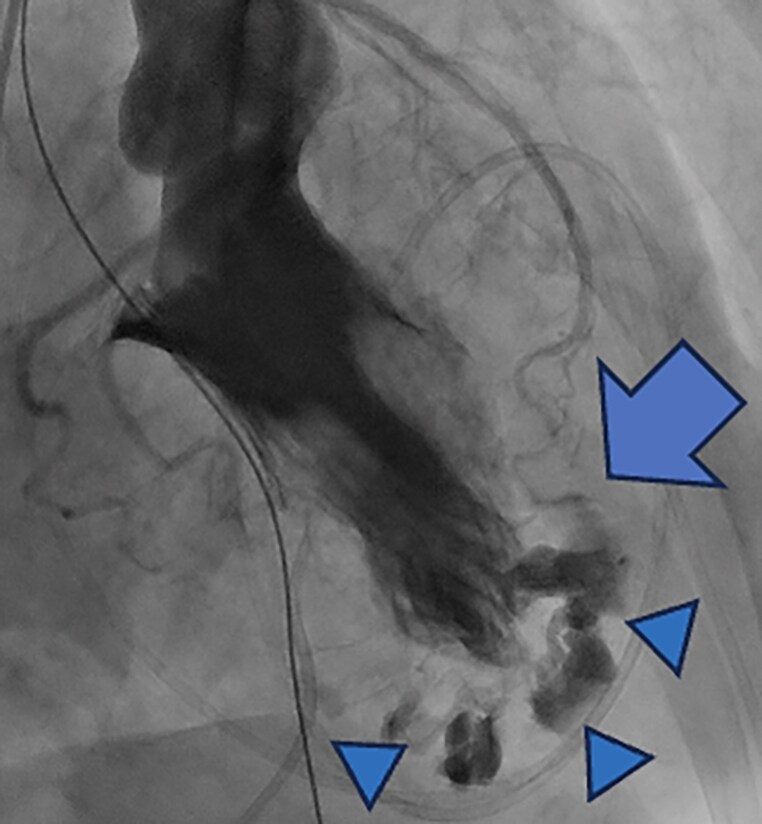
Video 2: left ventriculography. Left ventriculography revealed prominent contrast extravasation into the subepicardial fat tissue at the apex (arrowhead) and further leakage into the pericardial space (arrow), establishing the diagnosis of left ventricular free wall rupture. Video_3: intraoperative findings The patient underwent emergent surgery. After connecting the ECMO cannulas to the cardiopulmonary bypass (CPB) circuit, a median sternotomy was performed. Upon opening the pericardium, a large volume of dark, non-clotted blood was encountered. A supplemental venous cannula was placed via the right atrial appendage. Intraoperative inspection revealed a subepicardial haematoma at the left ventricular apex, but no active bleeding or discrete pericardial rent was identified. After temporary weaning from CPB and repeat inspection, no definite rupture site was seen. These findings suggest that a blow-out–type rupture had evolved to an oozing lesion. Two sheets of TachoSil® were applied to the apical region, and the patient was successfully weaned from CPB with intra-aortic balloon pump support.’ Video_4: postoperative CT findings. Postoperative contrast-enhanced computed tomography (CT) showed no evidence of re-rupture.
